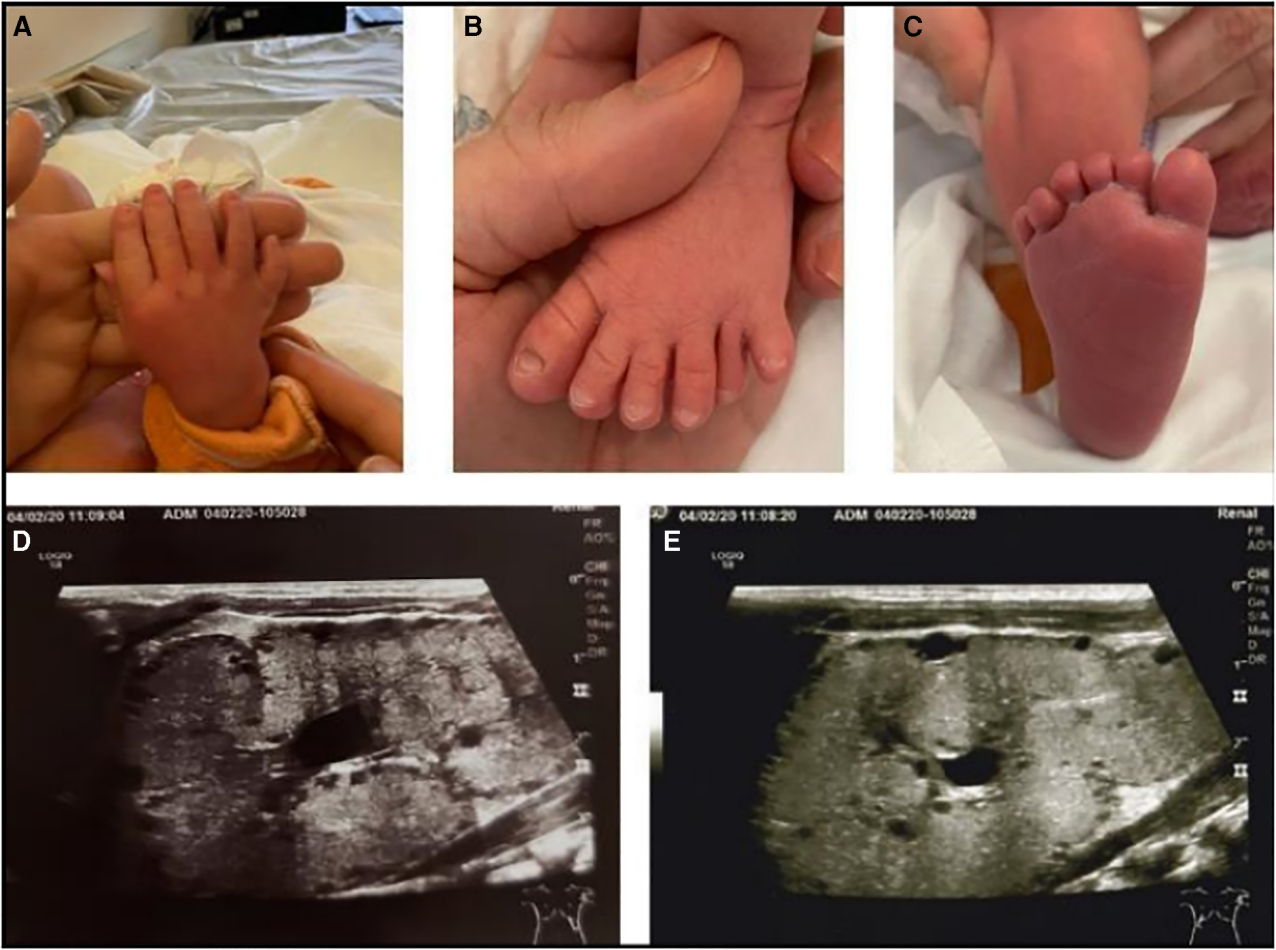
(A), hexadactyly of the right hand; (B), hexadactyly of the right foot; (C), hexadactyly of the left foot; (D,E), longitudinal ultrasound section through the left and right kidney.
